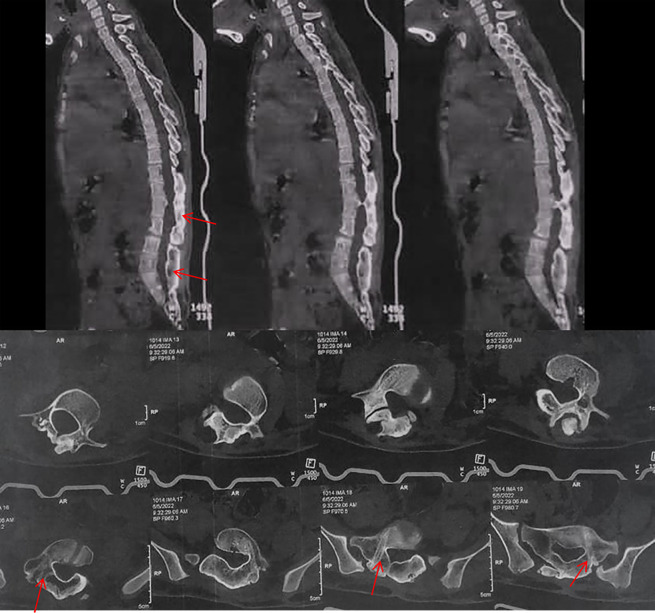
Axial and Sagittal CT cuts showing autofusion in a patient following growing rods removal, arrows pointing to the fusion mass and fused facets in the axial cuts.
